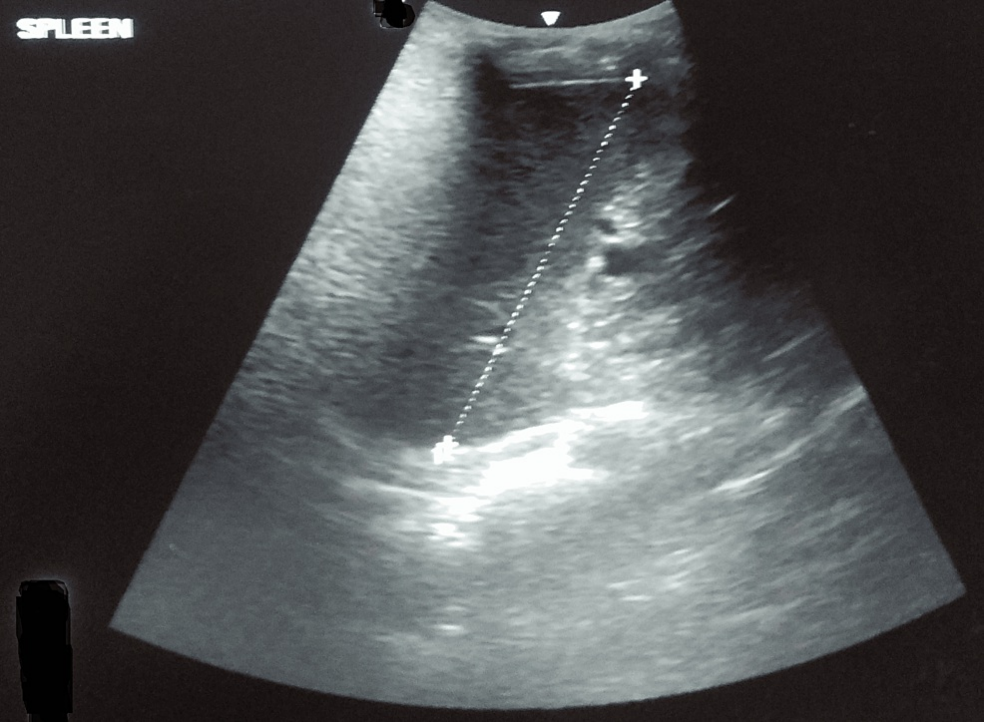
Latest ultrasonography showing a well-defined cystic lesion in the spleen
